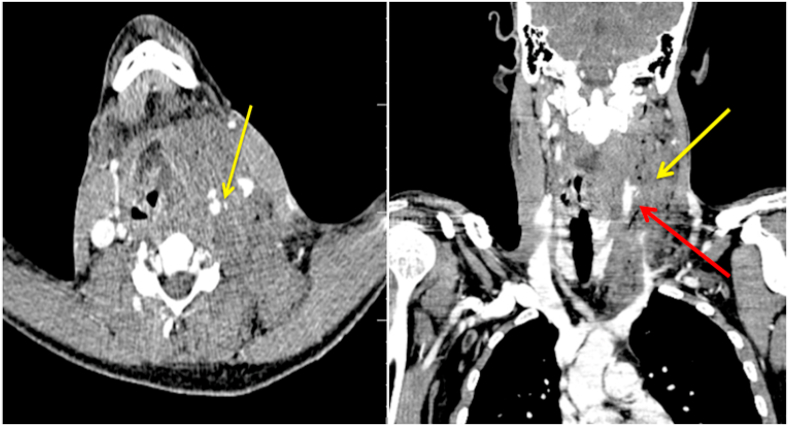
Right side CT Angiography coronal view shows Hematoma (yellow arrow), extravasation of contrast (Red arrow) and left side axial view shows extravasation of contrast and defect. (For interpretation of the references to color in this figure legend, the reader is referred to the Web version of this article.)
